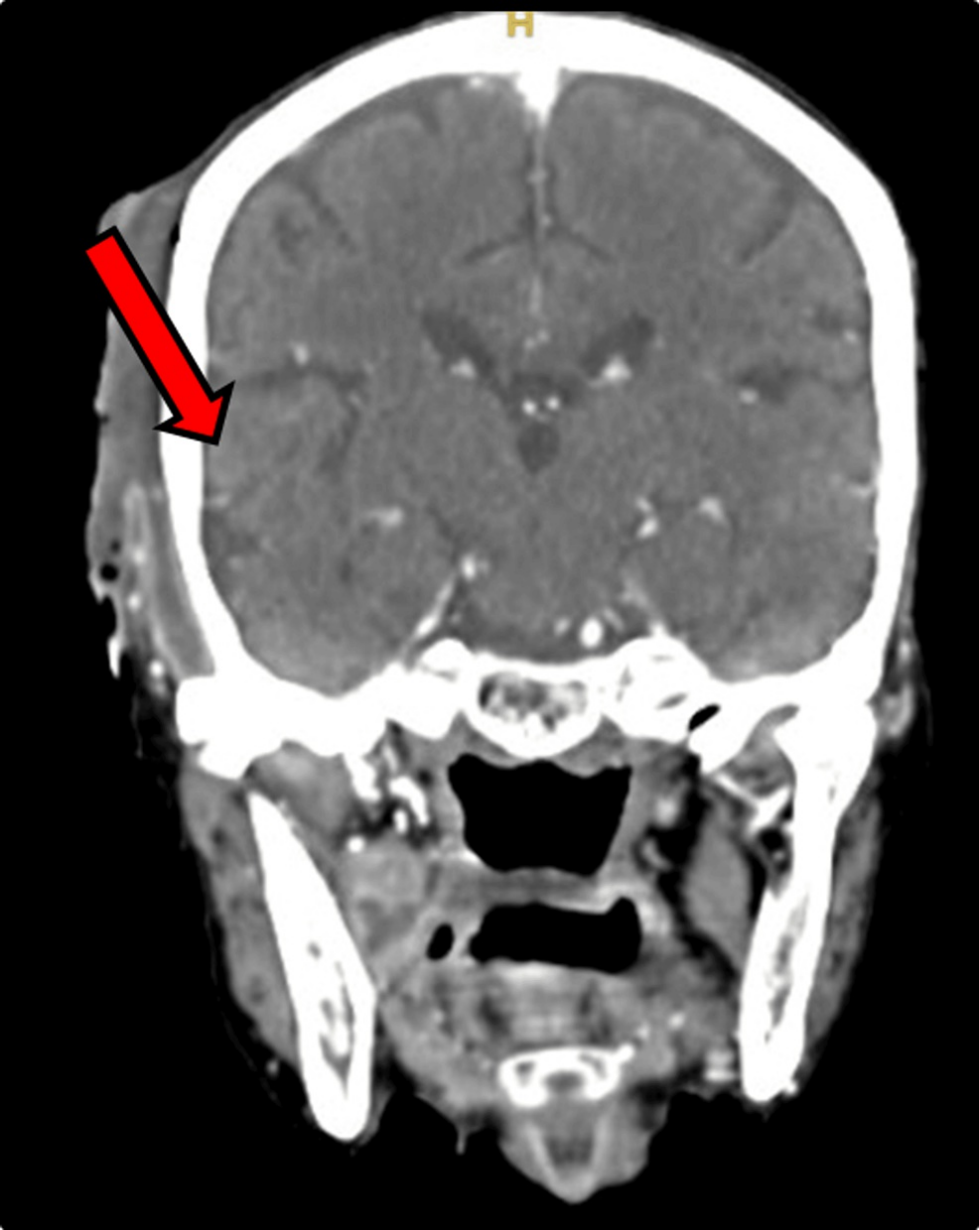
Coronal Section of Contrast-Enhanced Computed Tomograph (CECT) Head There is evidence of peripherally enhancing collection with internal enhancing septations within noted over the right frontoparieto temporal regions with multiple air density foci within (coronal section).
